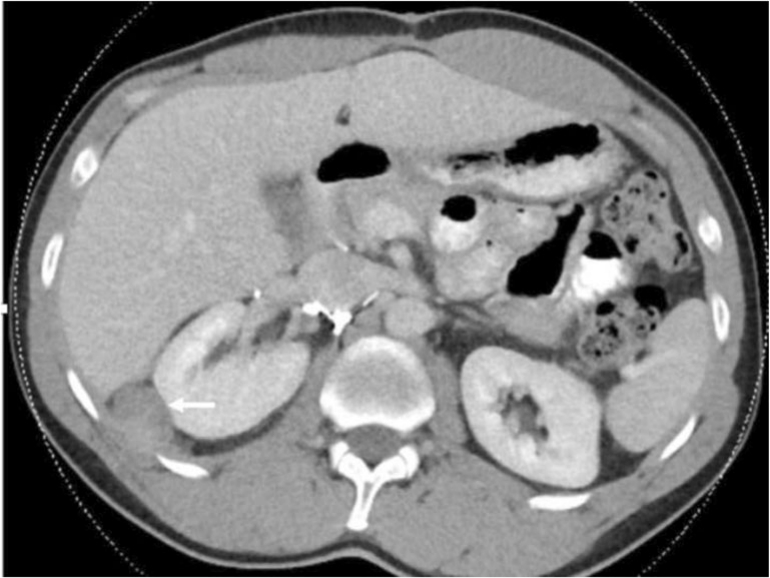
Axial portal venous phase CT shows a homogenous soft tissue metastasis in the right perinephric space, slightly indenting the adjacent kidney (*arrow*). The primary tumour, an adrenal carcinoma, had been excised previously (see clips dorsal to the inferior vena cava)
